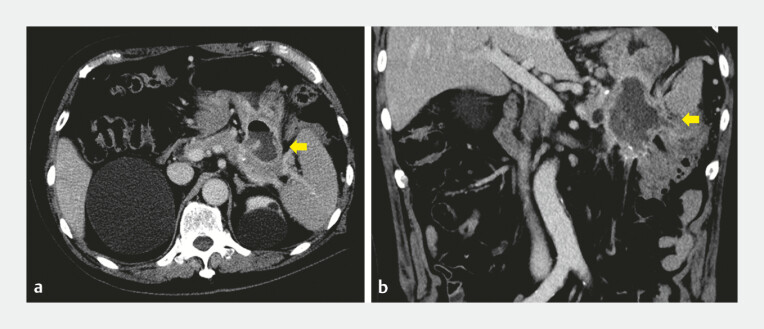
Computed tomographic images showing walled-off necrosis adjacent to the stomach. Axial (
